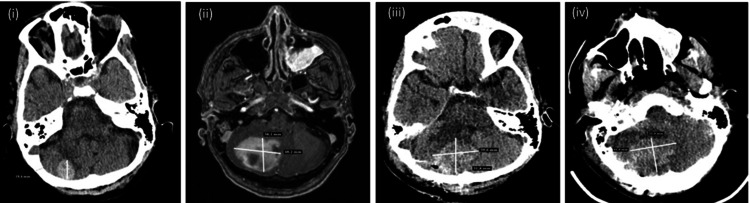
Pre and post-transplant imaging (i) Day 15, Pre-transplant: CT brain shows a 2 cm hyperdense lesion in the right cerebellar hemisphere with neighboring vasogenic edema, suspicious for hemorrhagic metastasis vs hematoma. (ii) Day 30, Pre-transplant: MRI brain shows a significant interval increase in the size of the hemorrhagic right cerebellar lesion, measuring approximately 3.8 x 4.8 x 2.6 cm, with increased surrounding vasogenic edema. (iii) Day 35, Post-craniotomy, Pre-transplant: CT brain shows postsurgical changes of right suboccipital craniotomy with an interval increase in size and density of a right posterior fossa hematoma, measuring approximately 3.1 x 4 x 3.2 cm. (iv) Day 37, Post-transplant: CT brain shows a grossly unchanged posterior fossa hematoma, measuring approximately 3.2 x 4.2 x 3.3 cm.
